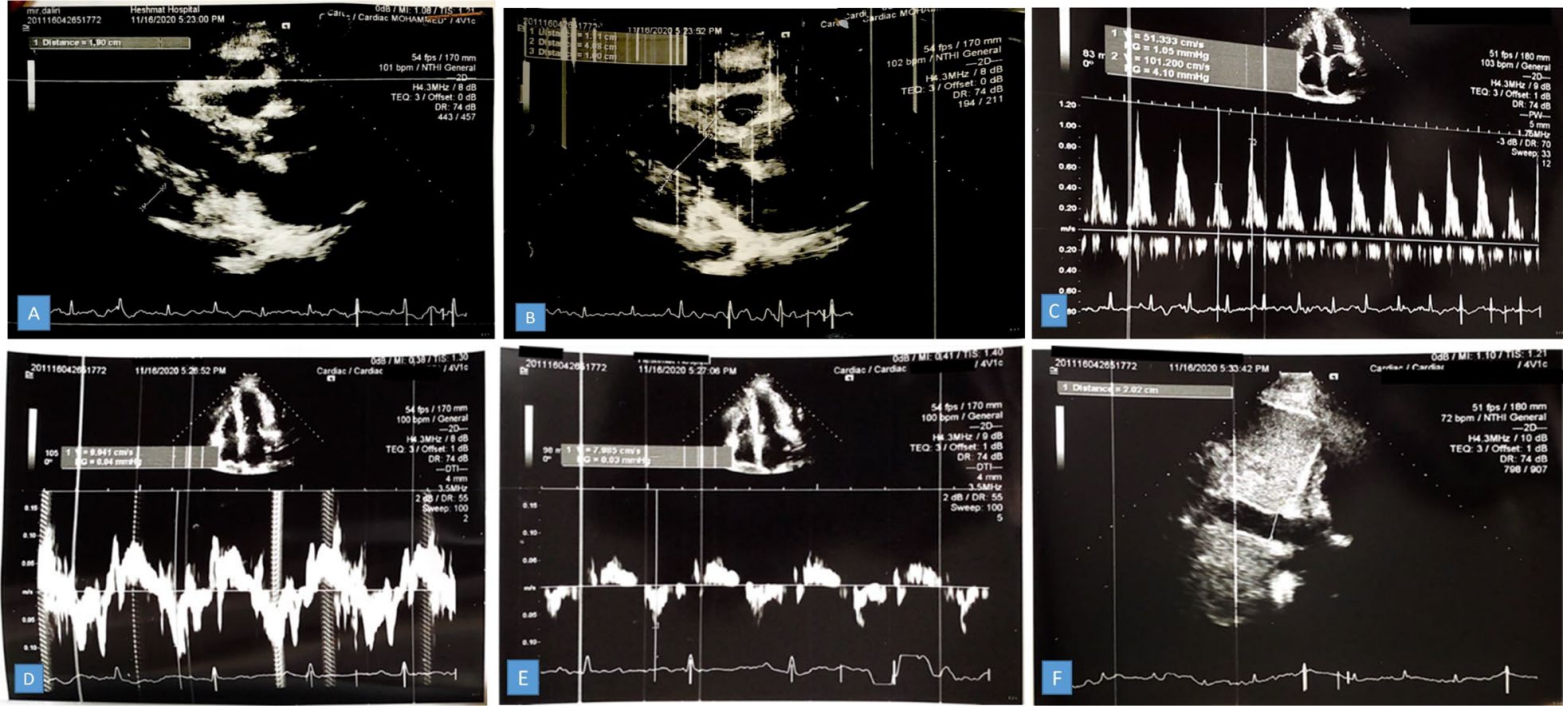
Echocardiography demonstrating Thickened pericardium and effusion in parasternal long-axis view (A, B), Exaggerated respiratory variation at the level of Mitral Valve (C), Annulus Reversus (D, E), normal IVC (20 mm) with reduced respiratory variation (F)
